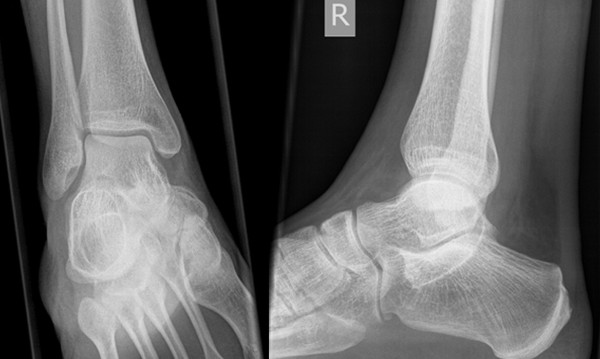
Conventional x-rays antero-posterior (left) and lateral (right) of the patient after trauma. No fracture or osseous lesion was found.
